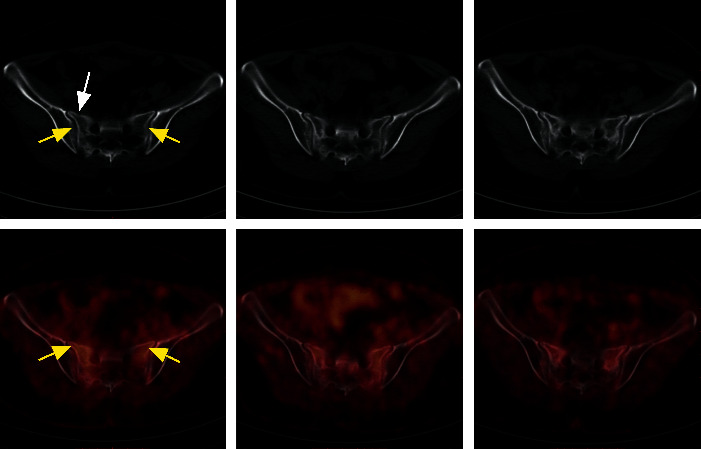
A 55-year-old woman who underwent pelvic radiotherapy for cervical cancer developed hip pain at 12 months after radiotherapy. Diffuse, mild, linear FDG uptake (left SUVmax, 3.2; right SUVmax, 3.0) parallel to the sacroiliac joint in the bilateral sacral ala (yellow arrow) was found in the fused axial image, while the low-dose CT image showed high density and a very small right anterior sacral fracture line (white arrow). Follow-up FDG PET/CT at 3 months after diagnosis of SIF. The fused image showed a slight increase in metabolism (left SUVmax, 3.3; right SUVmax, 3.5), and the low-dose CT image showed an increased bone density and high-density range. After 9 months of follow-up, the range of high density and hypermetabolism decreased; the metabolic intensity decreased (left SUVmax, 2.3; right SUVmax, 2.2), but the bone density increased.
